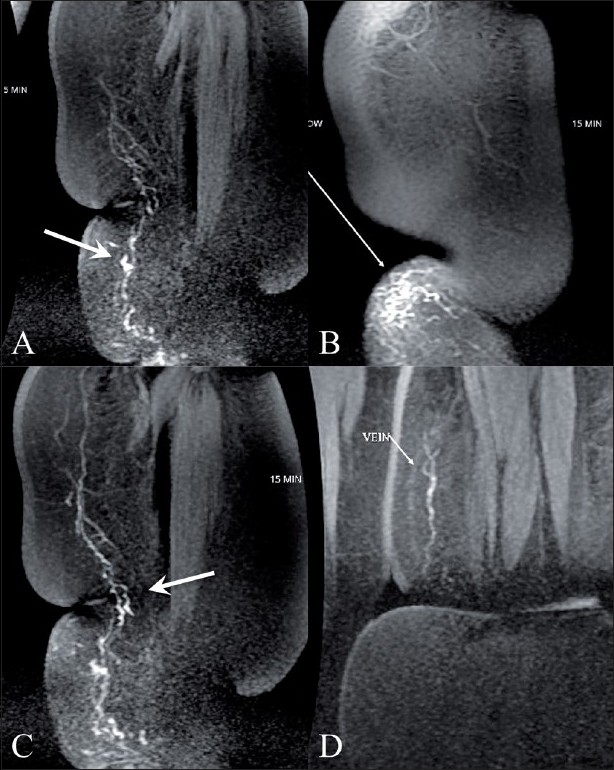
3D spoiled gradient-echo MRI images obtained at 5 min (A,B), 15 min (C) and 35 min (D) shows the lymphatics (arrows in A,C) with dermal backflow (arrow in B) and faint visualization of the veins at 35 min (arrow in D)
